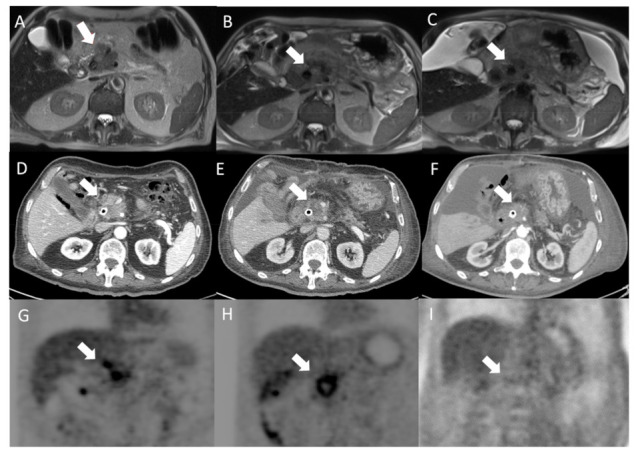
Head pancreatic cancer patient treated with fixed geometry. Before treatment, the arrow identifies the lesion on the HASTE T2-W sequence (A); after treatment the lesion (arrow) shows non-significant differences in signal intensity and size in (B) (one month after treatment) and in (C) (six months after treatment). In addition, non-significant difference on the CT study between pre- (D) and post-treatment was observed both after one month (E) and after six months by ECT (F). Instead, PET evaluation between pre- (G) and post-treatment detected a reduction of glucose uptake both after 1 month (H) and after 6 months (I) by ECT. According to data, the patient, after one month and after six months, was classified to be in stable disease by ECT.
